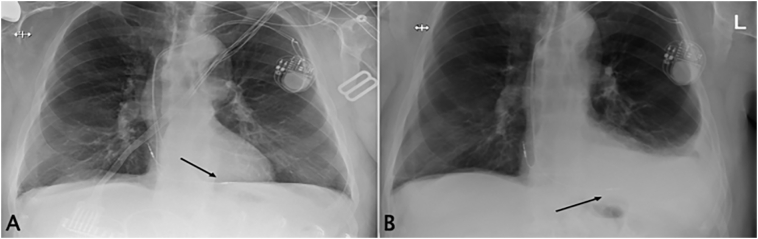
Chest radiograph confirming RV lead placement immediately after pacemaker placement (left panel; A) and when the patient returned to the hospital with pleuritic left chest pain (right panel; B); no lead migration was noted, but a large pleural effusion was present.
